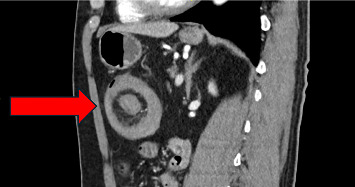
Sagittal CT of intussusception, displaying the characteristic “target sign” or “donut” morphology.
